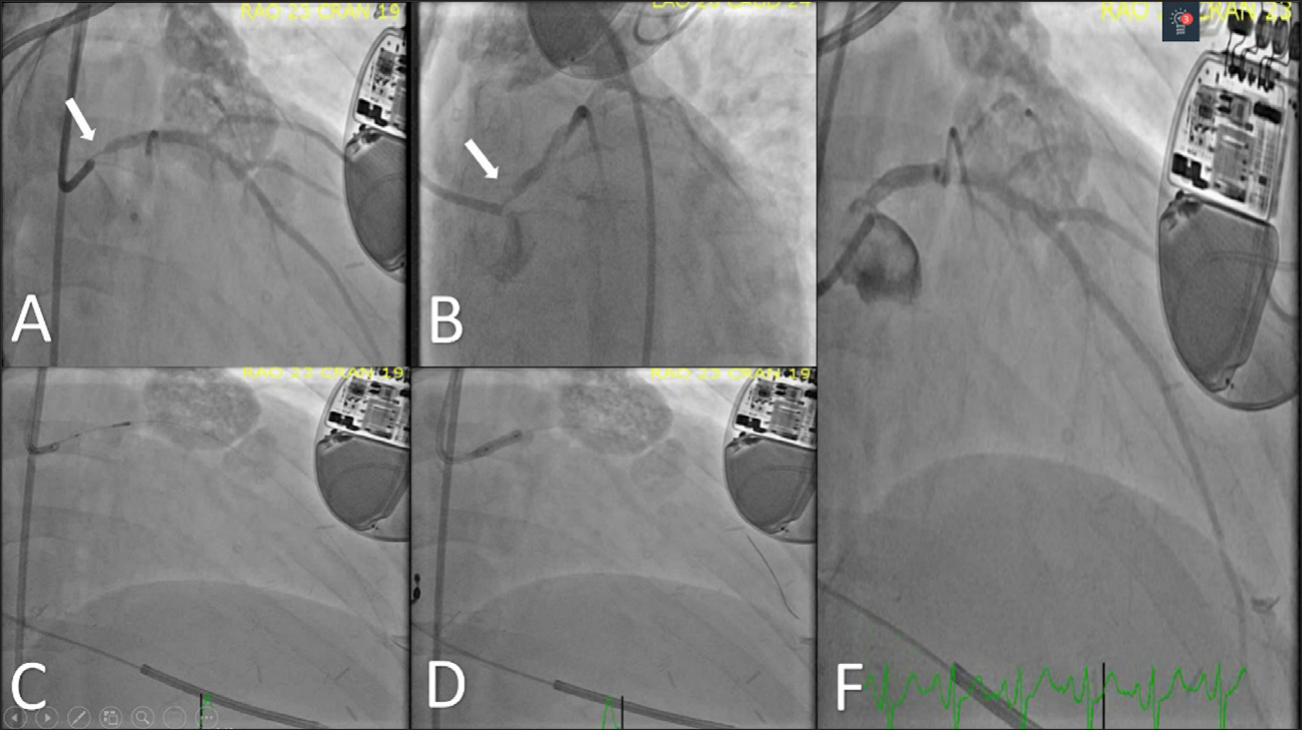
Percutaneous coronary intervention of a severe ostial left main coronary artery stenosis. (A, B) Severe (95%) stenosis of the ostial left main coronary artery; (C) intravascular ultrasound to assess lesion and plan stent sizing; (D) deployment of a 3.5 × 12 mm Xience (pre-dilated with a compliant balloon and postdilated with a noncompliant 4.0 × 12 mm balloon); (F) final angiography showing 0% residual stenosis with TIMI 3 flow distally without complications. TIMI: Thrombolysis in Myocardial Infarction Score
